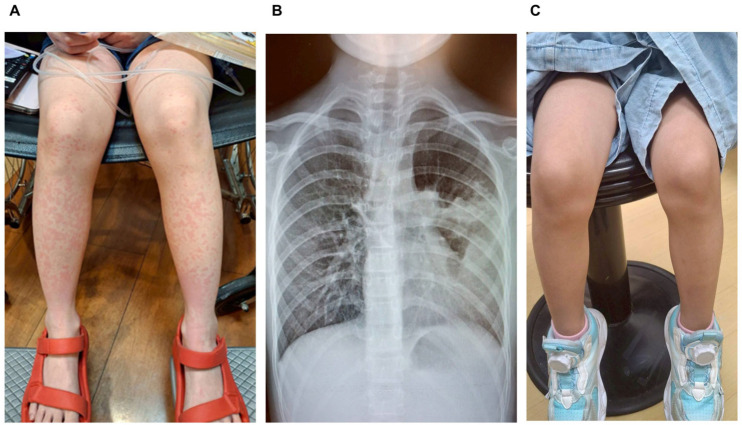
(A) Representative photograph of the severe, widespread, pruritic urticaria affecting the face, trunk, and extremities, shown here on the lower extremities, before Omalizumab treatment. (B) The Chest X-ray shows a prominent consolidation in the left mid and lower lung lobes, characterized by the presence of an air bronchogram sign. (C) After Omalizumab treatment, the urticaria resolved rapidly the following day.
